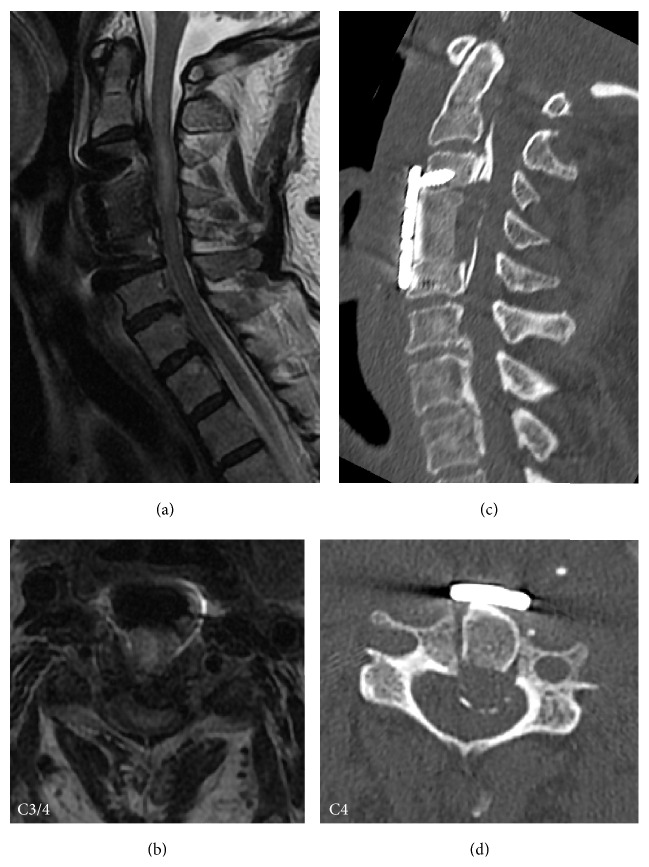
MRI and CT images taken at onset of C5 palsy. MRI (a, b) and CT imaging (c, d) obtained immediately after onset of C5 palsy showed the intramedullary T2 high signal change and spinal cord enlargement from C2 vertebral body level to C4 vertebral body level (a, b) without any graft dislodgement or implant malposition (c, d).
